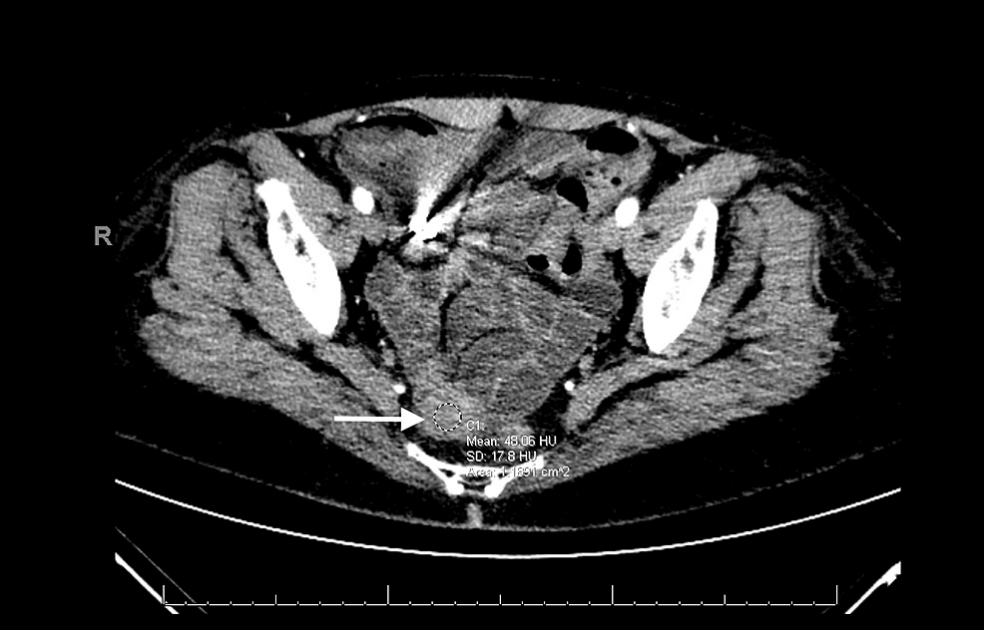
CTA abdomen and pelvis White arrow shows mid-ileal 3.3 × 2.6 × 2.7 cm^3^ mass favoring a GIST
